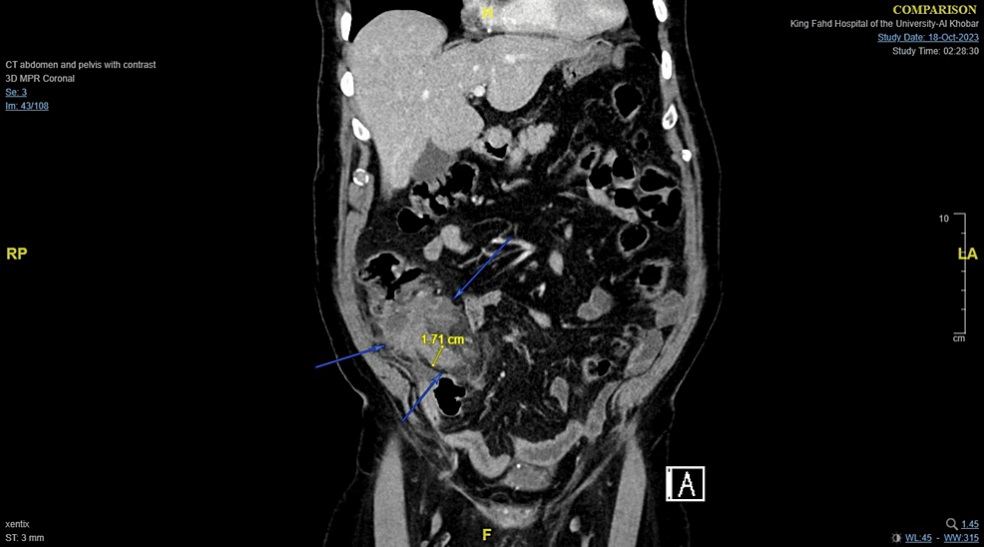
CT scan of the abdomen showing thickened dilated appendix reaching 1.71 cm with free fluid and fat stranding. The adjacent terminal ileum and cecum are significantly inflamed with enlarged regional lymph nodes.
